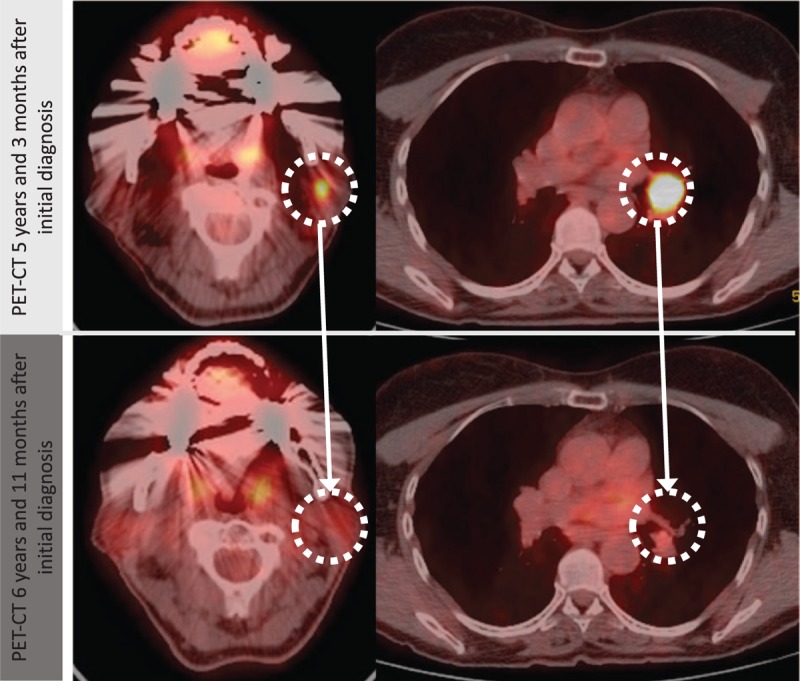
PET-CT of the first diagnose of a regional (cervical) and hilar lymph node metastases and the follow-up scan where the metastases are in complete remission.
